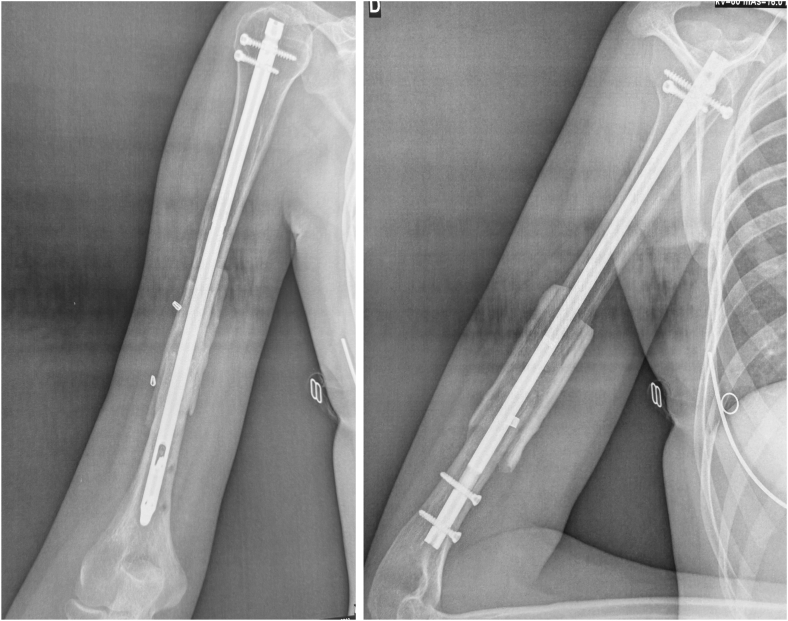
Anteroposterior and lateral radiographs of the humerus obtained at 3-month follow-up demonstrating early allograft integration following treatment of a humeral shaft nonunion with exchange intramedullary nailing and circumferential cortical strut allograft augmentation (Sarcophagus technique).
